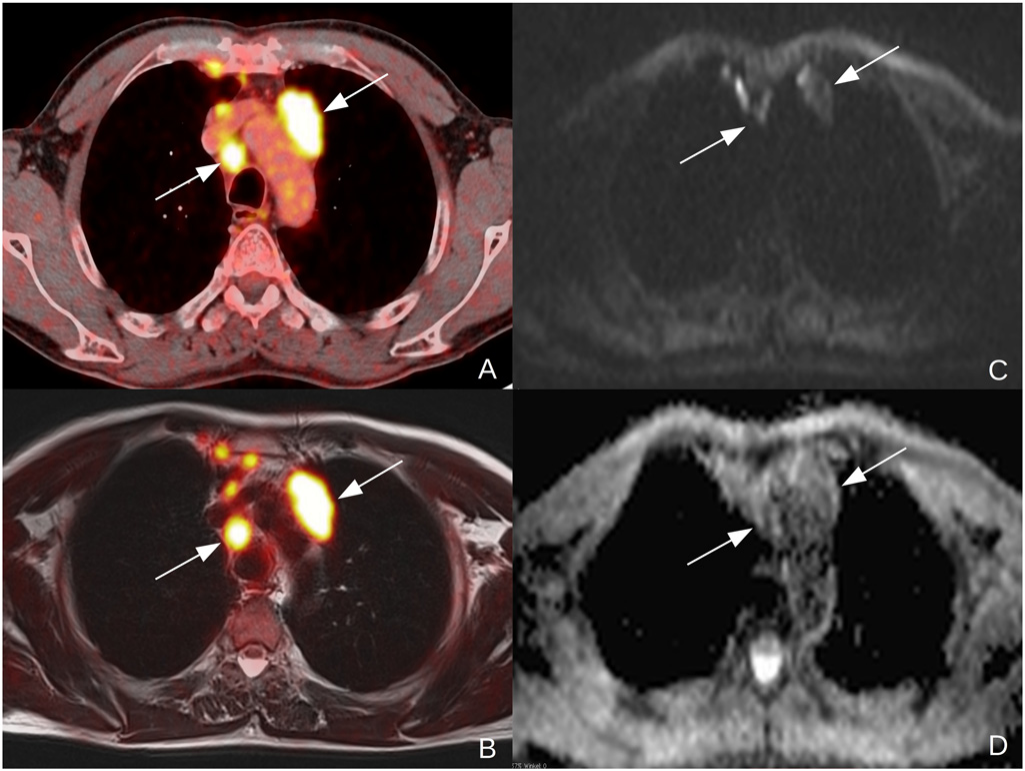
57y old male patient suffering from NSCLC (tumor stage IIIb, squamous cell carcinoma, unknown tumor grading) Clearly malignant lymph nodes to the left of the aortic arch and right to the trachea in a contrasted enhanced, fused PET/CT-image (A) and in a T2-weighted, fused PET/MR-image (B). The lymph nodes are clearly depicted in the diffusion weighted b1000-image (C) and show low ADC-values in the corresponding, monoexponential ADC-map. The additional, smaller lymph nodes in the anterior mediastinum were not visible in DWI (C) and were therefore excluded from further analysis.
